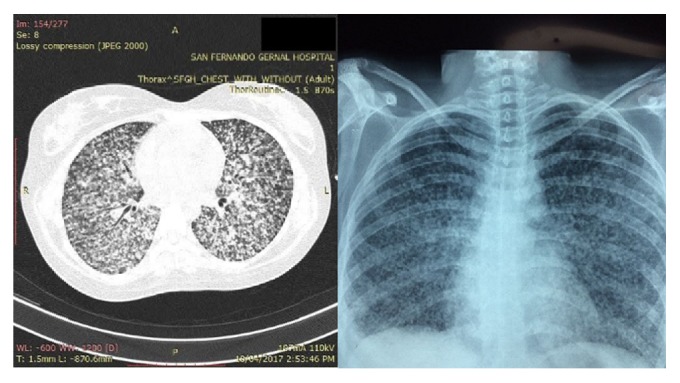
CT scan and CXR of patient showing bilateral miliary opacification.
